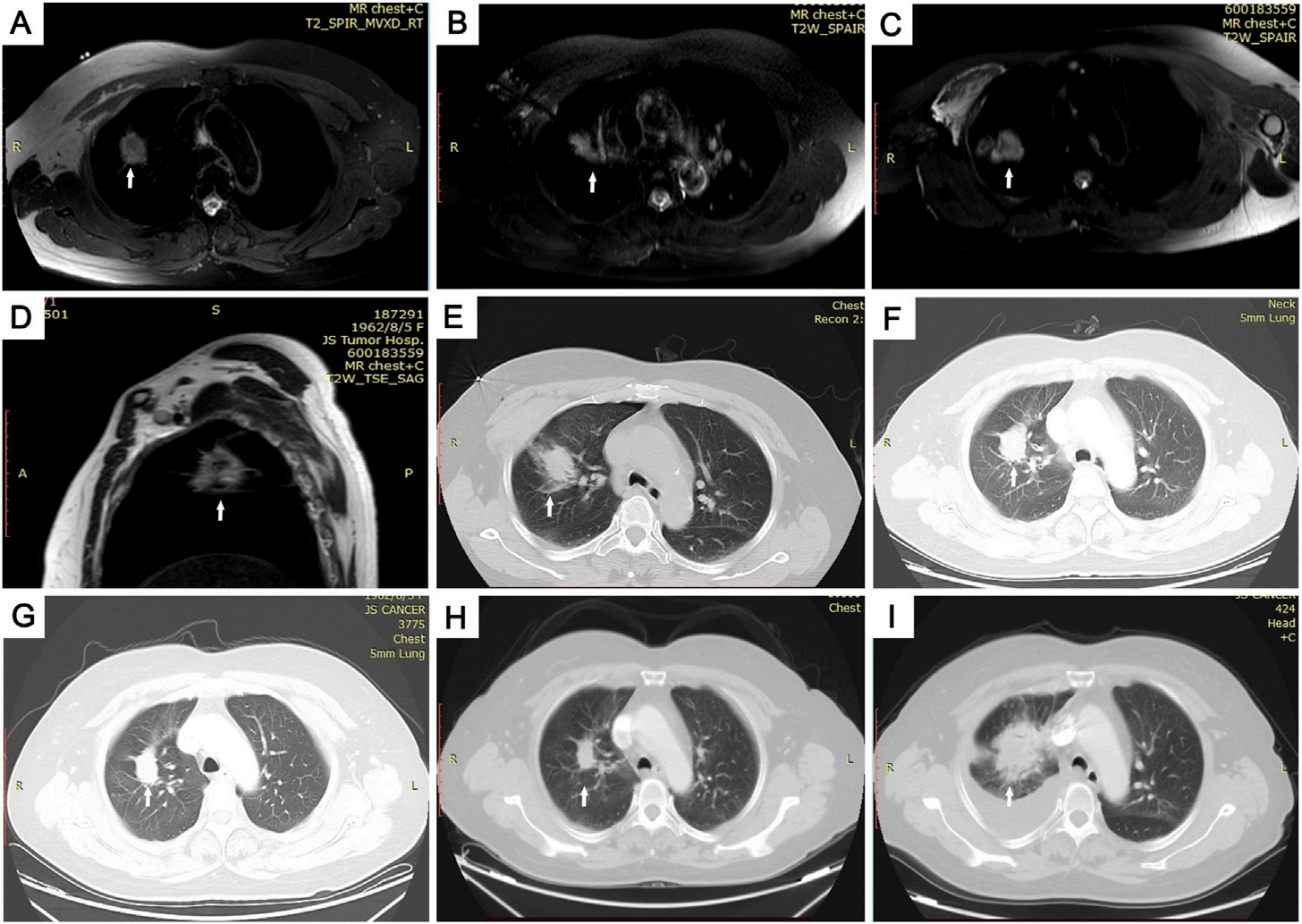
Images of the MRI-guided MWA procedure and follow-up in 59-year-old woman with pulmonary adenocarcinoma in superior lobe of right lung. (A) Skin markers were applied to locate the needle insertion site under magnetic resonance imaging (MRI) guidance. (B) Guided by magnetic-resonance T1WI image, 16G-ablation antenna was punctured to the center of the tumor lesion. (C–E) After two 8-min ablation cycles, we achieved a satisfactory ablation area, which is clearly seen in axial- (C) and sagittal- (D) phase images, and a large area of ground glass covers the entire tumor on the lung window of CT I. (F–I) Computed tomography (CT) images of follow-up. Follow-up CT scans at 3 (F), 6 (G), and 12 (H) months show significantly shrinking, and follow-up CT scan at 21 months (I) showed the tumor had increased in size.
